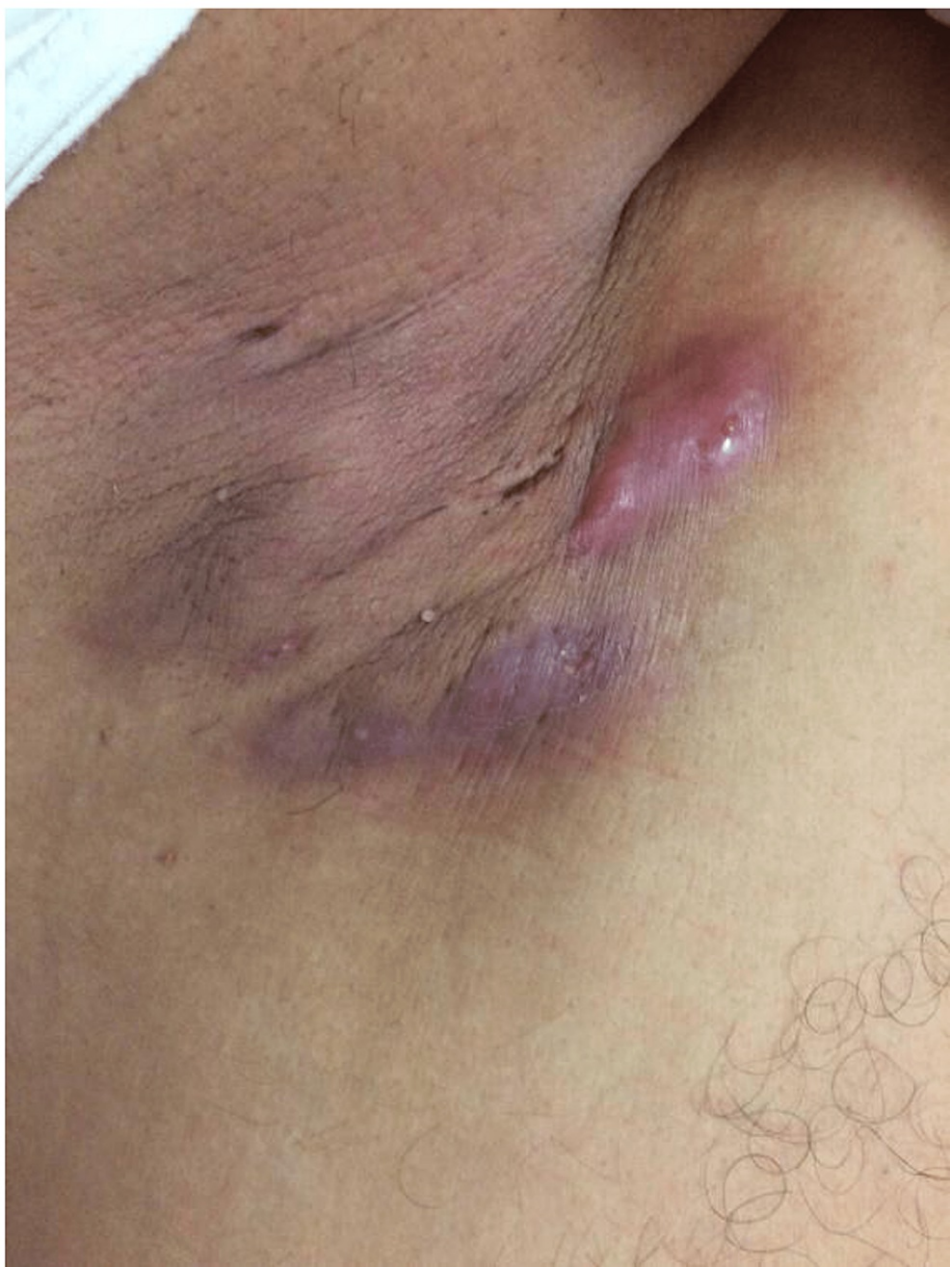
HS Multiple erythematous lumps in the axilla Source: Fluorine-18 Fluorodeoxyglucose PET/CT Images of Hidradenitis Suppurativa Mimicking Metastasis in a Patient with Small Cell Lung Carcinoma by O. Ekmekcioglu, K. Sonmezoglu, Cureus, licensed under CC BY 4.0. HS: Hidradenitis suppurativa
